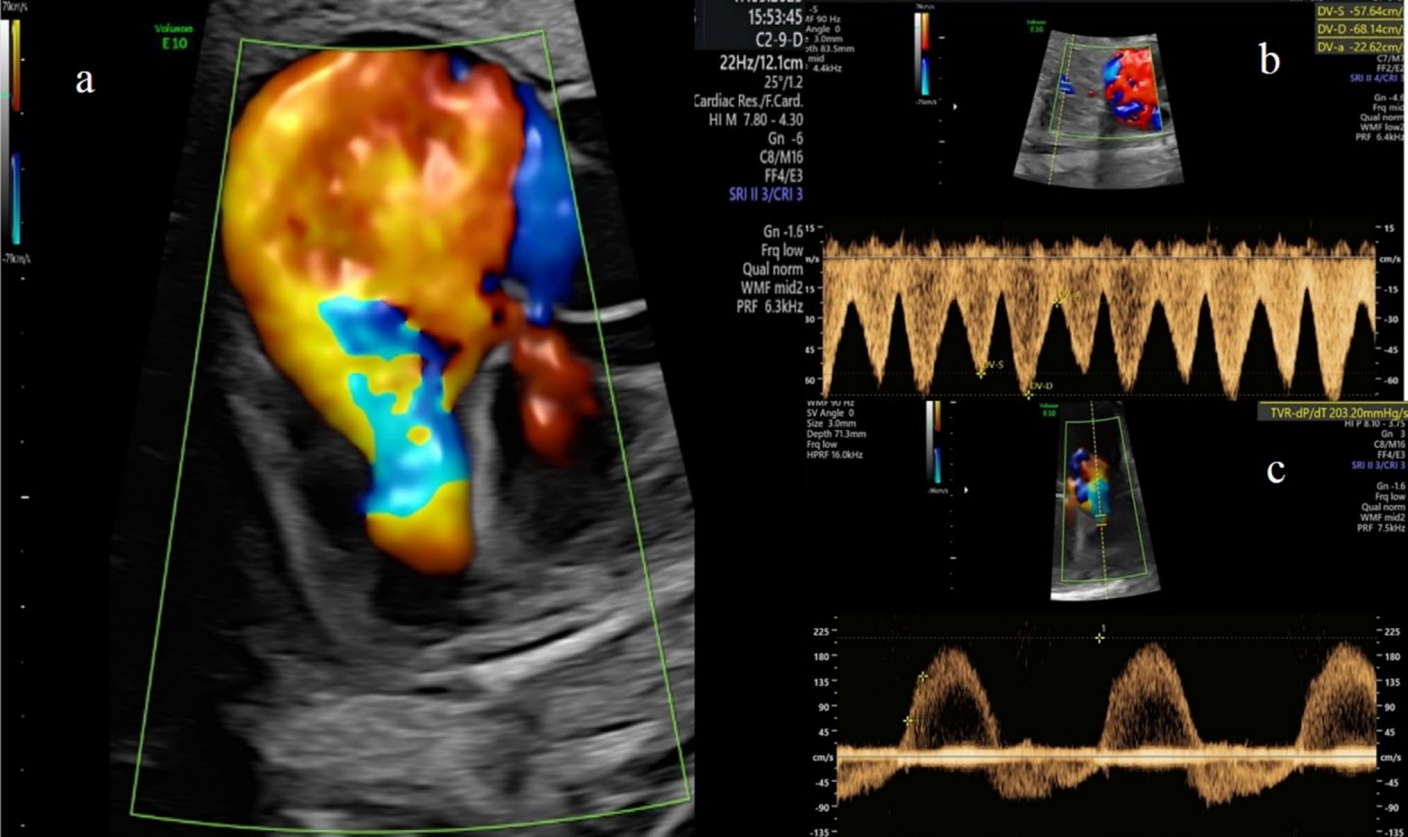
Case 2 a Ebstein anomaly at 33 + 0 weeks of gestation, demonstrating massive dilated right atrium with a regurgitant jet arising from the middle of the right ventricle on color Doppler imaging. Color contours appears as “hot air balloon”. b Pulsed-wave Doppler of the ductus venosus in the same patient demonstrates a decrease of systolic peak velocity compared to diastolic flow (S < D) and deep v-wave, indicating severe tricuspid insufficiency. c Impaired right ventricular function was demonstrated by low peak systolic velocity of 210.8 cm/s and calculating the dP/dt parameter of only 203.2 mmHg/s in tricuspid regurgitation
